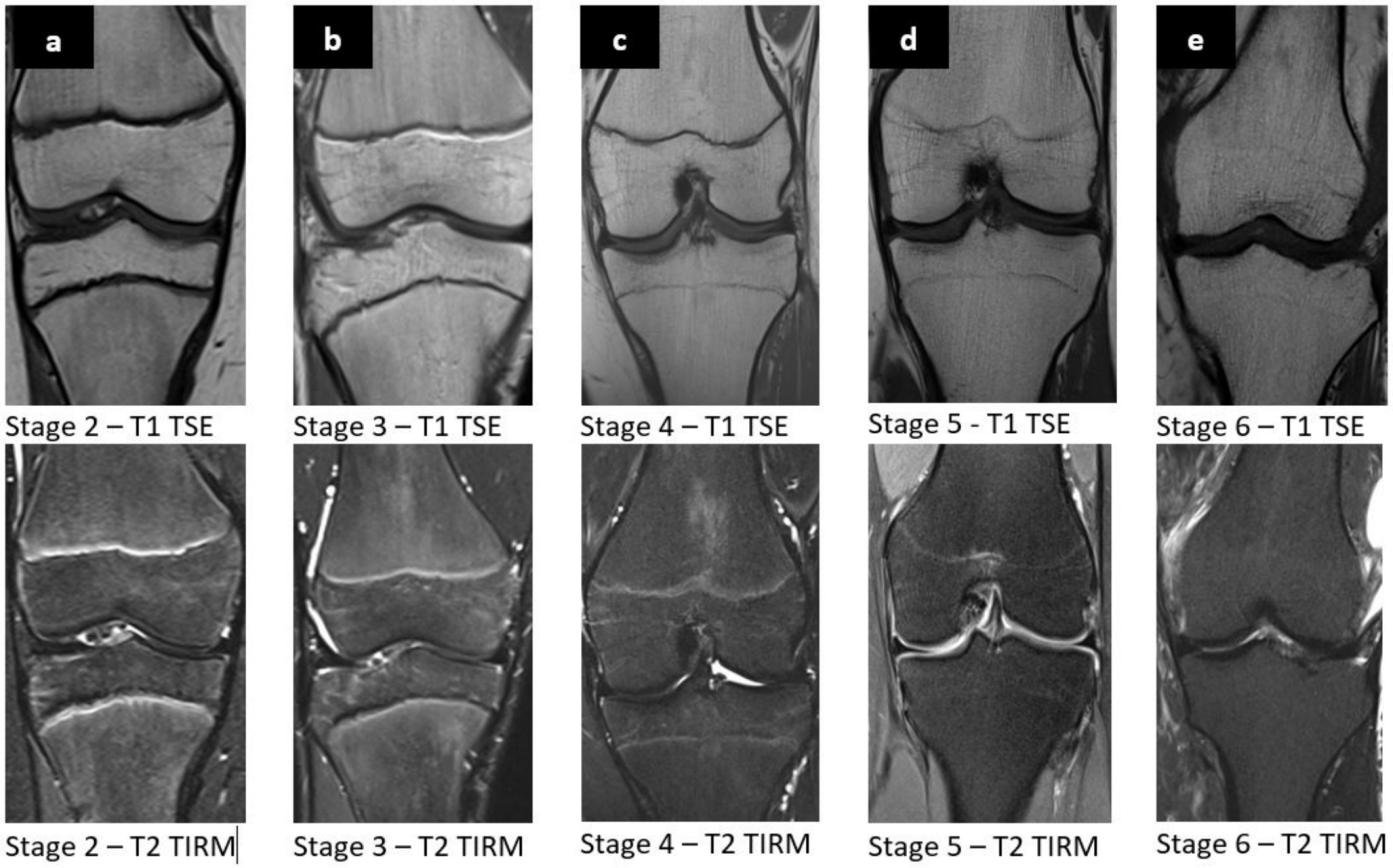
Coronary MRI images in T1-TSE sequence (first row) and T2-TIRM sequence (second row). a: distal femur and proximal tibia in in stage 2 of a 10-year-old male patient; b: distal femur and proximal tibia of a 14-year-old male patient in stage 3; c: distal femur and proximal tibia of a 14-year-old female patient in stage 4; d: distal femur and proximal tibia of a 17-year-old female patient in stage 5; e: distal femur and proximal tibia of a 24-year-old female patient in stage 6; MRI: Magnetic Resonance Imaging, TSE: Turbo Spin Echo, TIRM: Turbo Inversion Recovery Magnitude
